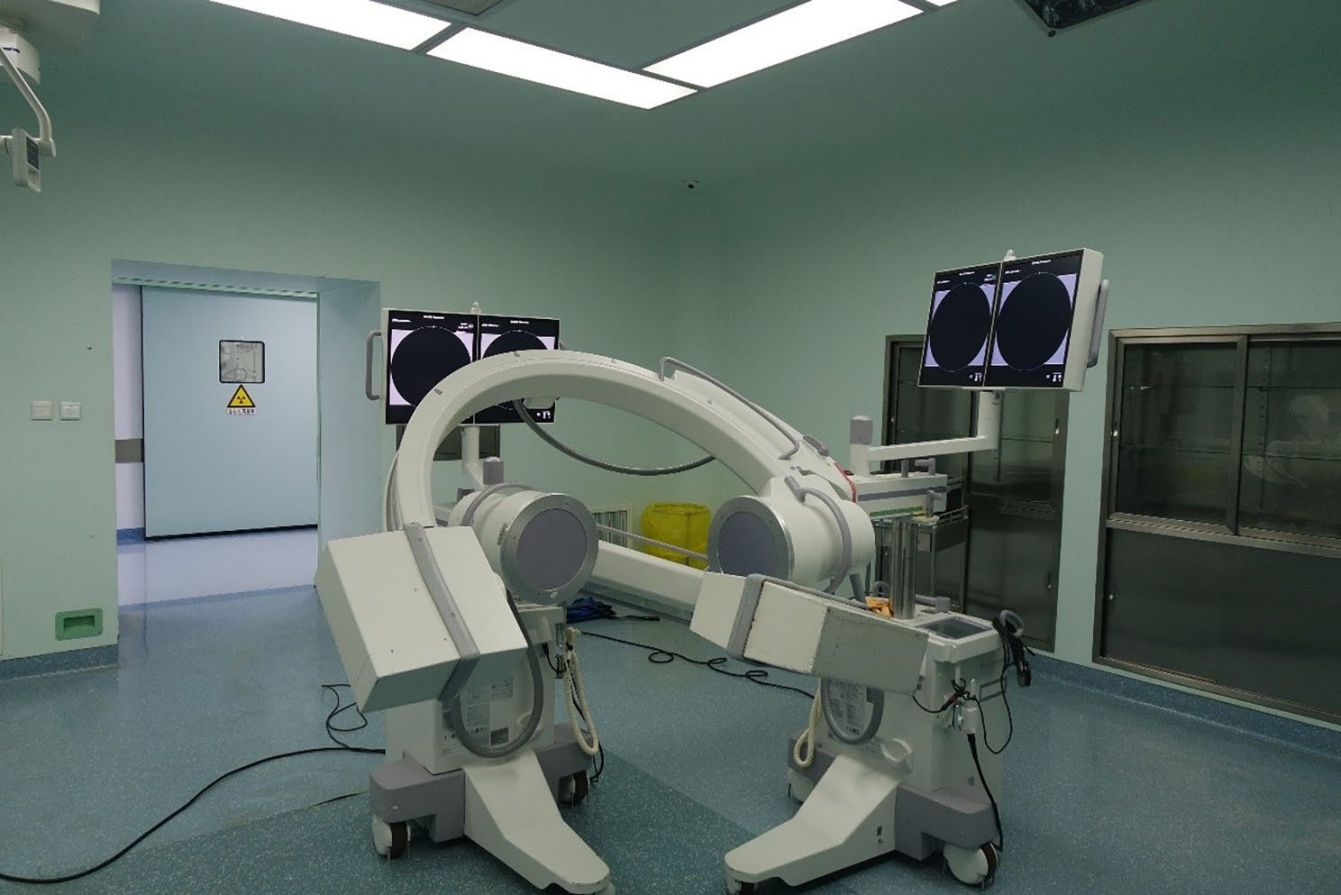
Dual fluoroscopic imaging system: The DFIS system was composed of two mobile X-ray machines placed perpendicular to each other
